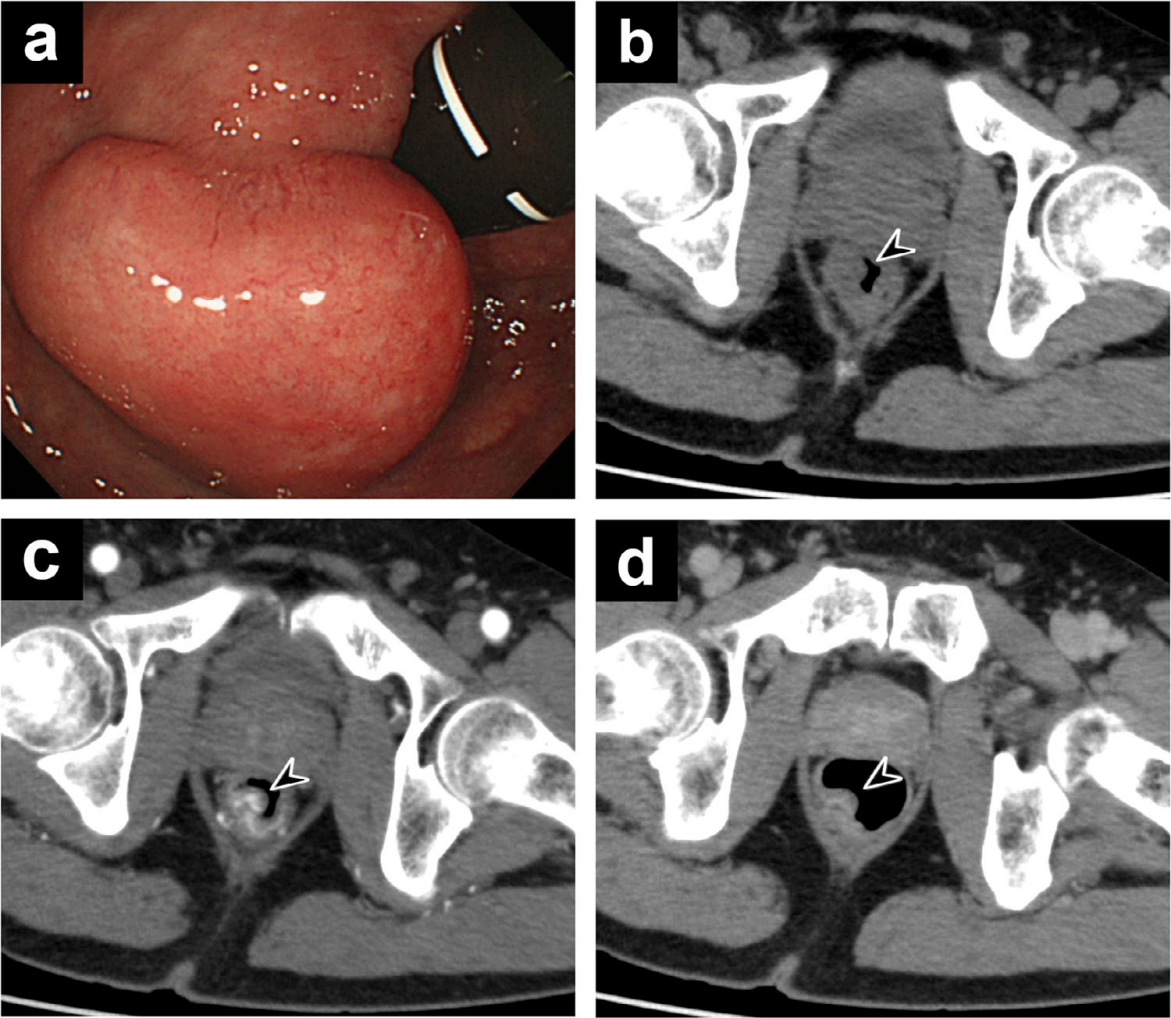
(a) Colonoscopy showing a submucosal tumor‐like protruding lesion in the lower rectum near the dentate line, with normal overlying mucosa. (b) Non‐contrast computed tomography showing a mass protruding into the lumen on the right side of the lower rectum (arrowhead). (c) Early‐phase contrast‐enhanced computed tomography demonstrating a hypervascular lesion with a cluster‐like vascular pattern (arrowhead). (d) Late‐phase image showing persistent contrast enhancement of the lesion (arrowhead).
